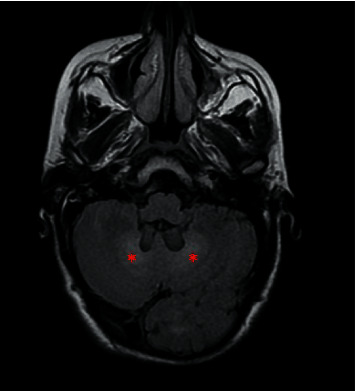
Axial image of brain MRI showing bilateral T2 hypersignal of the serrated nuclei (asterisks).
